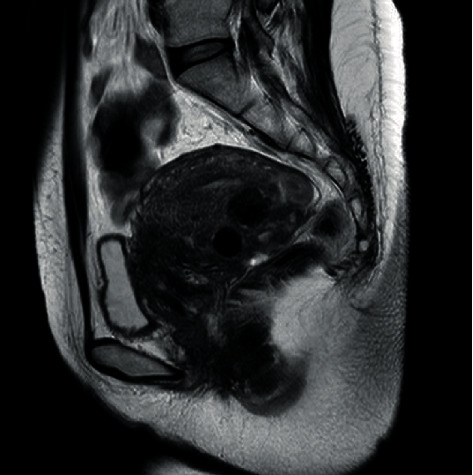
Magnetic resonance imaging was performed 2 years after uterine artery embolization. The leiomyomas grew smaller.
